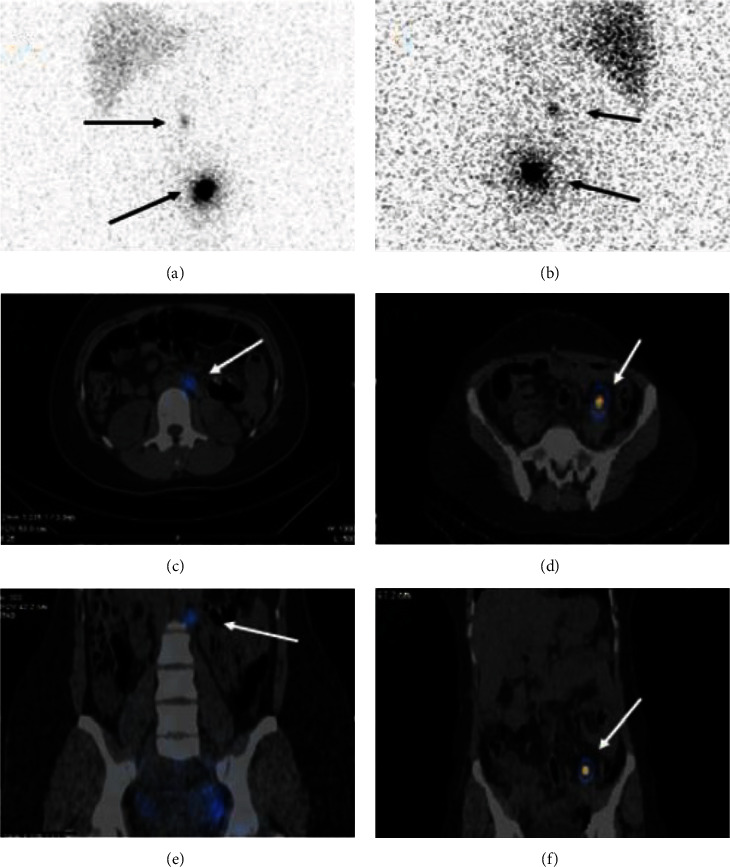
Planar lymphoscintigraphy in the (a) anterior and (b) posterior views show hotspots in the mid-abdomen and left pelvic side. SPECT/CT shows the exact location of these hotspots compatible with para-aortic and left internal iliac sentinel lymph nodes in the (c, d) transverse and (e, f) coronal views.
